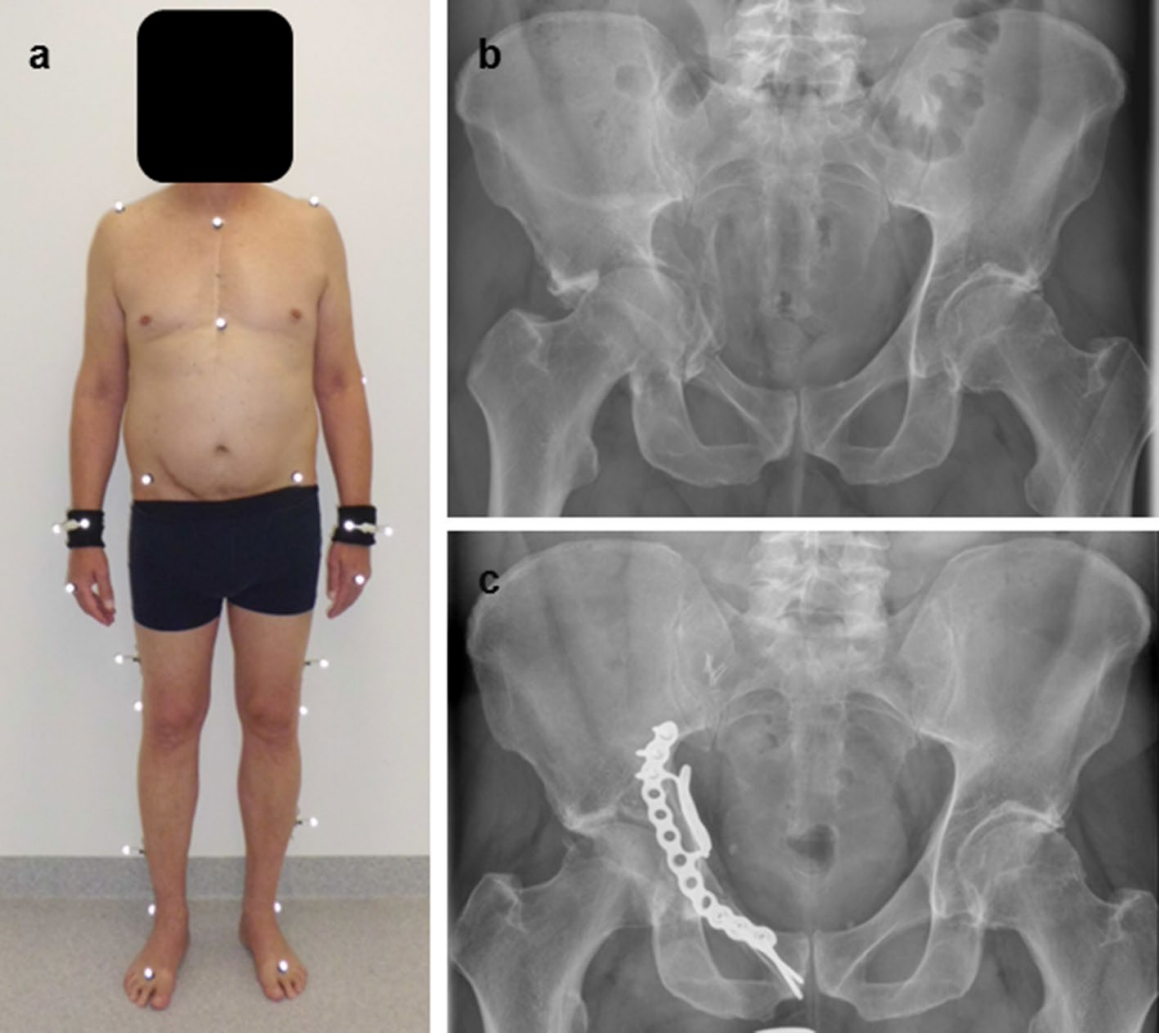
Patient equipped with retroreflective markers for biomechanical follow-up (a) following an acetabular fracture involving the quadrilateral plate on the right side treated using the pararectus approach (b, c). Postoperative radiograph shows the fracture reduced without any step or gap using an anatomically pre-contoured small fragment plate (Stryker PRO system, Stryker Corp., Kalamazoo, MI, USA)
